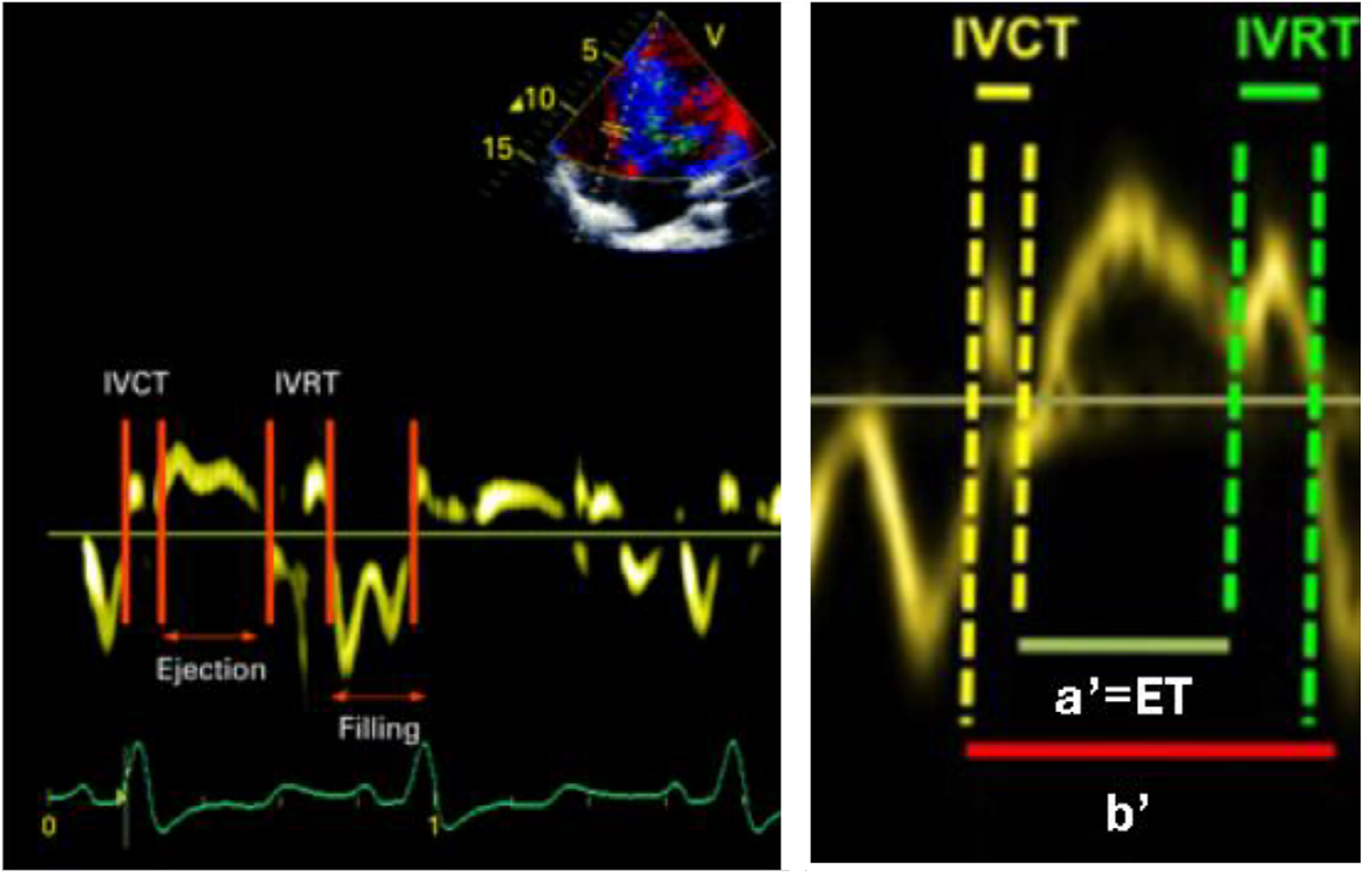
Tissue Doppler imaging (TDI) of the basal segment of the right ventricular free wall. Calculation can be done using the time intervals (left) or an alternative way(right).
